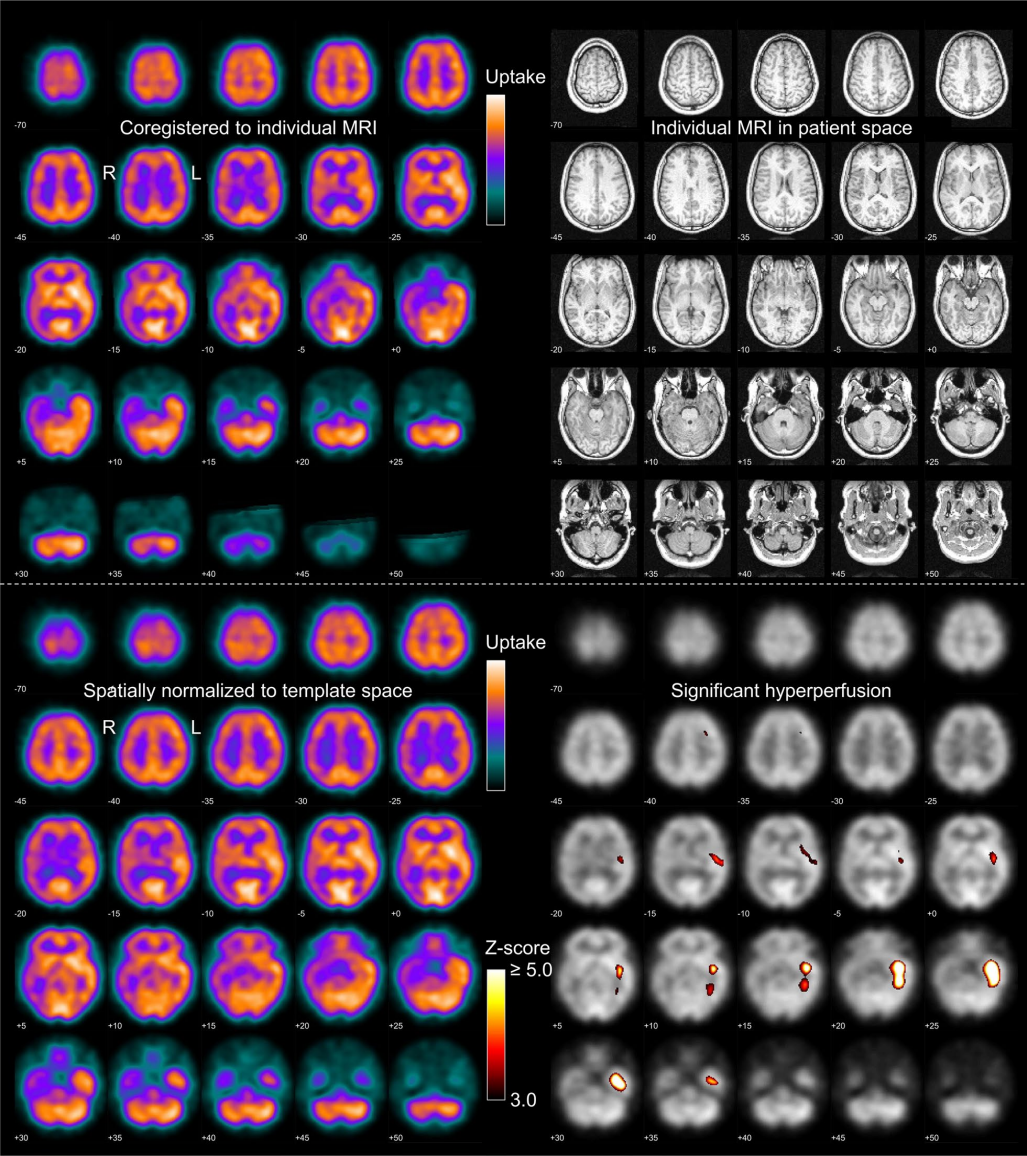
Standardized display for visual reading. The upper and lower parts were provided as page 1 and page 2 of a 2-page pdf-document (separate pdf-document for each patient). On the first page, the left side shows the patient’s ictal perfusion SPECT coregistered to the individual T1-weighted MRI shown on the right. On the second page, the left side shows the patient’s ictal perfusion SPECT after stereotactical normalization into the anatominal standard space, the righ side shows the statistical hyperperfusion map thresholded at a z-score of 3.0 and overlaid to the patient’s SPECT. This representative report is from a 16y old female with post-encephalitic lesion in the left temporal lobe. Ictal perfusion SPECT was performed with 500 MBq ^99m^Tc-ECD injected 19 s after electrical start of the seizure. The seizure continued for 41 s after the tracer injection. All three readers lateralized the SOZ in the left hemisphere and localized it to the left tempotal lobe, both with highest certainty. After surgical resection of the lesion, the subject was free of seizures (Engel IA) during the entire follow-up of 60 months
